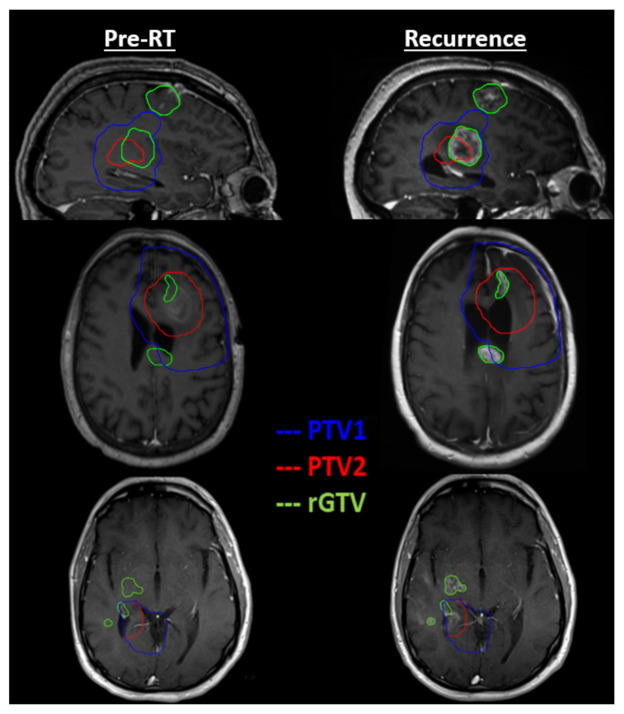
T1 post-contrast imaging in three patients, by horizontal row, with out of field recurrence in cohort 2. For each patient, the enhancing recurrence contour (rGTV) encompasses lesion that has spread outside the extent of radiation treatment targets PTV1 (guided by T2/FLAIR) or PTV2 (guided by T1w-CE).
